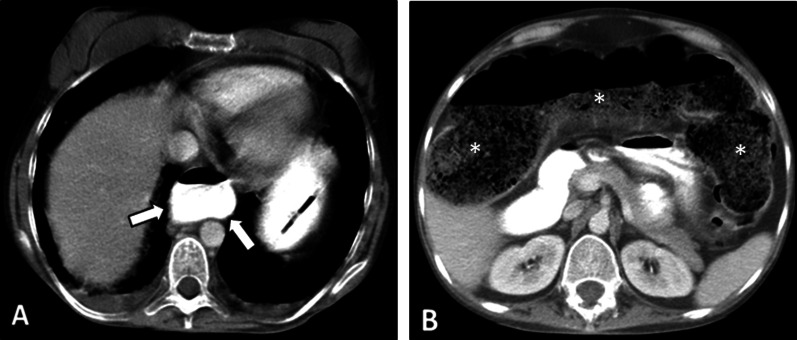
Colonic pseudo-obstruction in a 35-year-old female with known scleroderma presenting with constipation, nausea, vomiting, and abdominal distension. CT scan with IV/oral contrast was done. Axial CT image (A) demonstrates dilation of the distal esophagus (thick white arrows), a common finding in scleroderma. Axial CT image (B) reveals marked diffuse dilatation of colon (*) without obvious wall thickening or abrupt transition point, suggestive for colonic pseudo-obstruction (Ogilvie syndrome)
